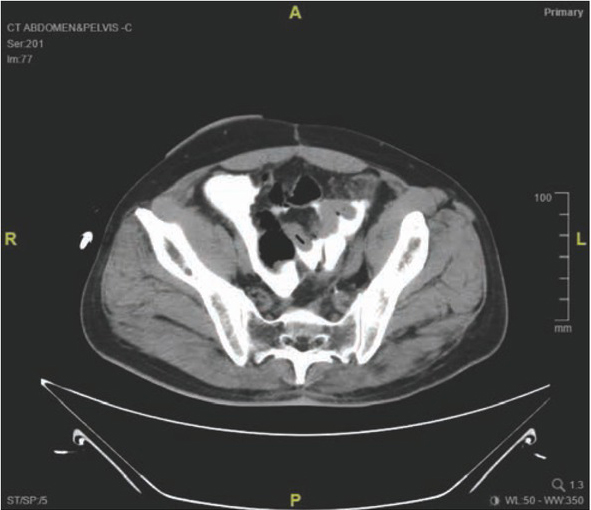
(Case 2) CT abdomen done eight hours after the distal loopogram, showed contrast pooling at the pelvis.
